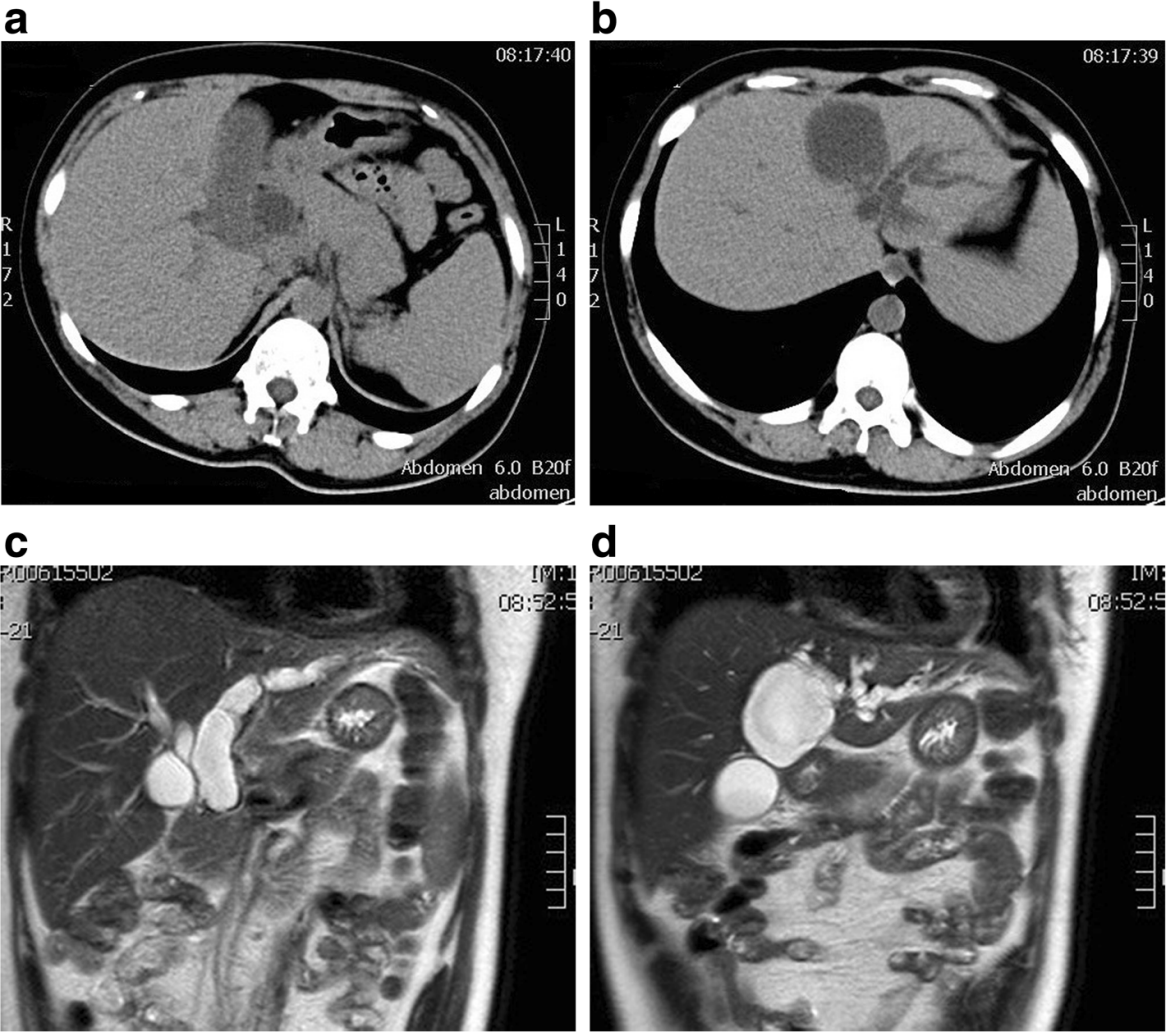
Imaging examination on admission: a Epigastric CT shows greatly ectatic common bile duct. b Epigastric CT shows greatly left hepatic duct. c MRCP shows slightly ectatic right hepatic duct, greatly ectatic left hepatic duct and common bile duct. d MRCP shows hepatic hilar region and enlarged gallbladder
